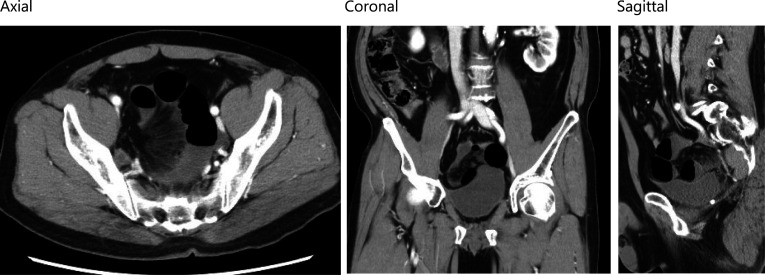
Contrast-enhanced CT scan of the case 2 showed closed-loop in the right pelvic cavity (left: axial, center: coronal, right: sagittal)
